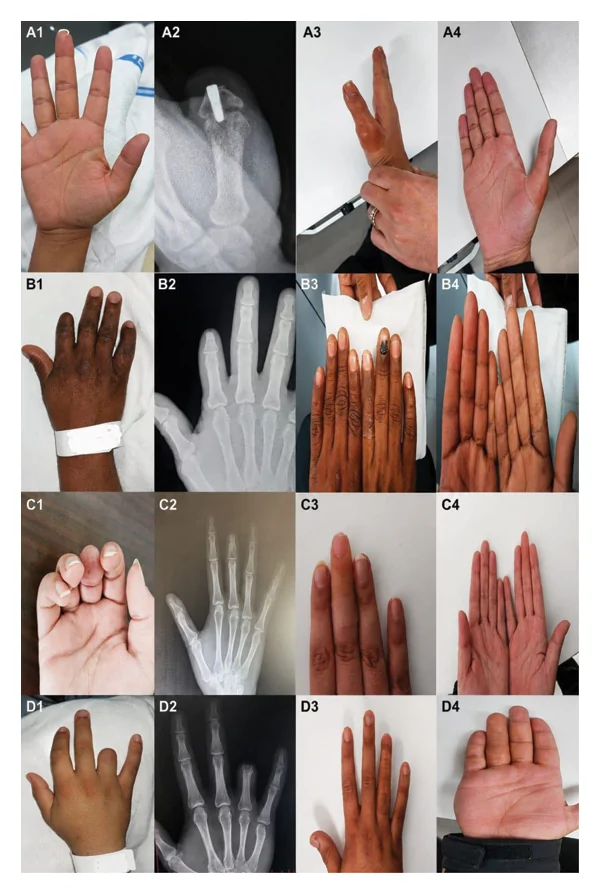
Preoperative clinical and radiological assessment and postoperative outcomes following silicone finger prosthetic rehabilitation. First row (A1–D1): preoperative clinical photographs of the affected hand. Second row (A2–D2): preoperative radiographs showing the level of bone amputation. Third row (A3–D3): postoperative clinical photographs showing the silicone prosthesis in situ (dorsal view of the hand). Fourth row (A4–D4): postoperative clinical photographs showing the silicone prosthesis in situ (palmar view of the hand).
